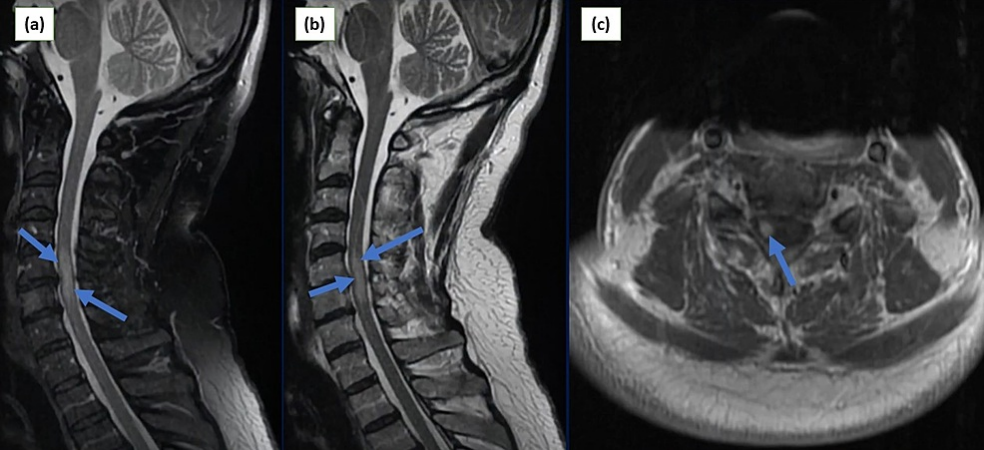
Cervical MRI images: (a) sagittal view demonstrating T1-weighted image with signal enhancement from C4-C6; (b) sagittal view showing T2-weighted image with hyperintense signals from C3-C5; (c) axial view of cervical spine demonstrating uniform enhancement at the level of C4. MRI: magnetic resonance imaging, C: cervical.
